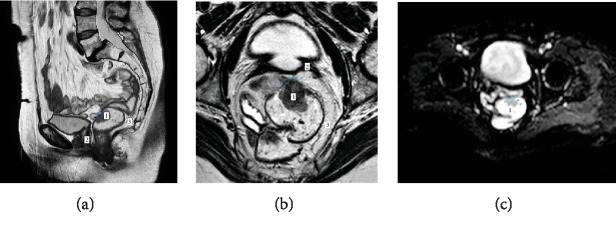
Radiologic data from a high-resolution pelvic MRI. Tumor of the lower rectum (1) situated on the anterior wall was observed on sagittal (a) and axial T2-weighted scans (b) with signs of mesorectal invasion (blue arrowheads) ranging up to 3 mm and no radiologic features of lymph node metastases (3). Vaginal stump and rectovaginal sept (2) had a significantly low intensity MR signal due to their fibrotic transformation. On a series of diffusion-weighted images (c), a significant delay of diffusion together with intense signaling on high b-factors was observed at the level of a tumor (blue arrowheads).
